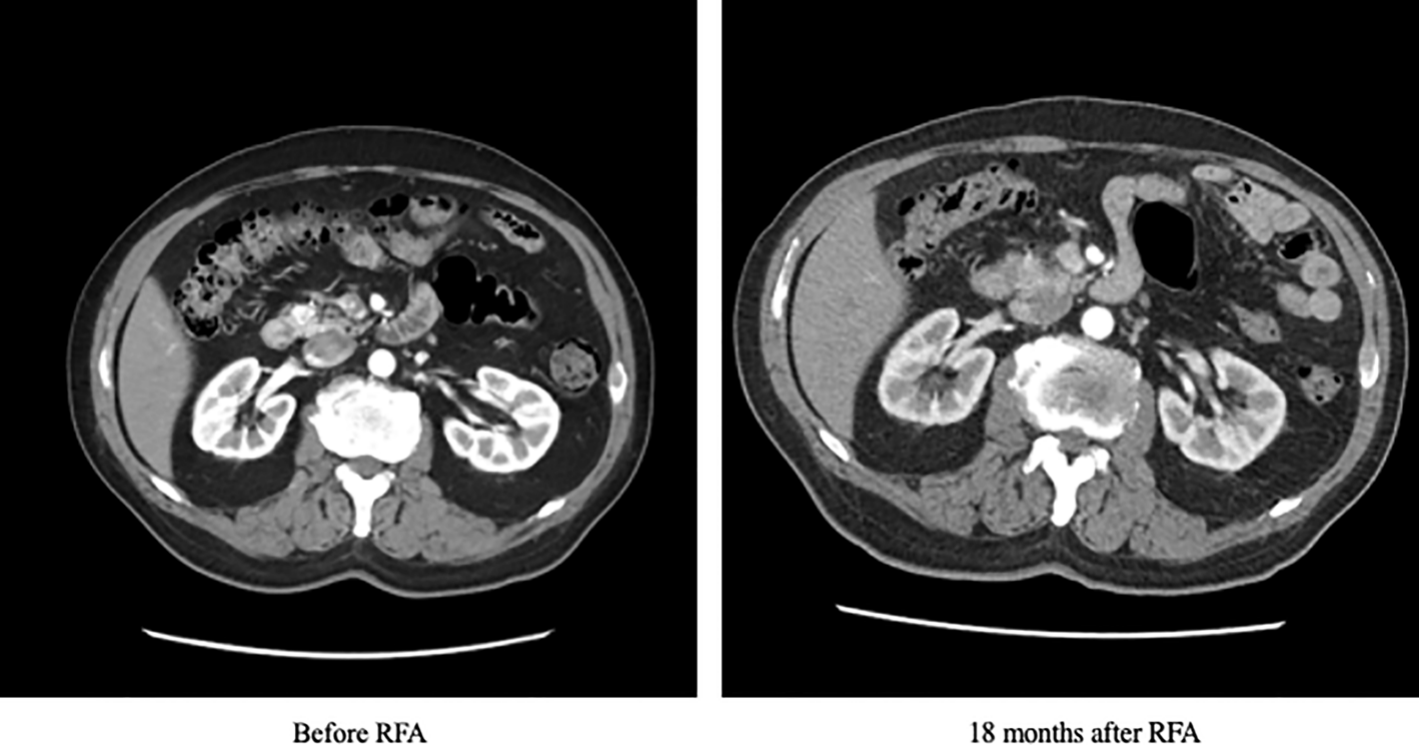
Contrast CT scan of the patient at baseline and 18 months after RFA. CT, computed tomography; RFA, radiofrequency ablation.
